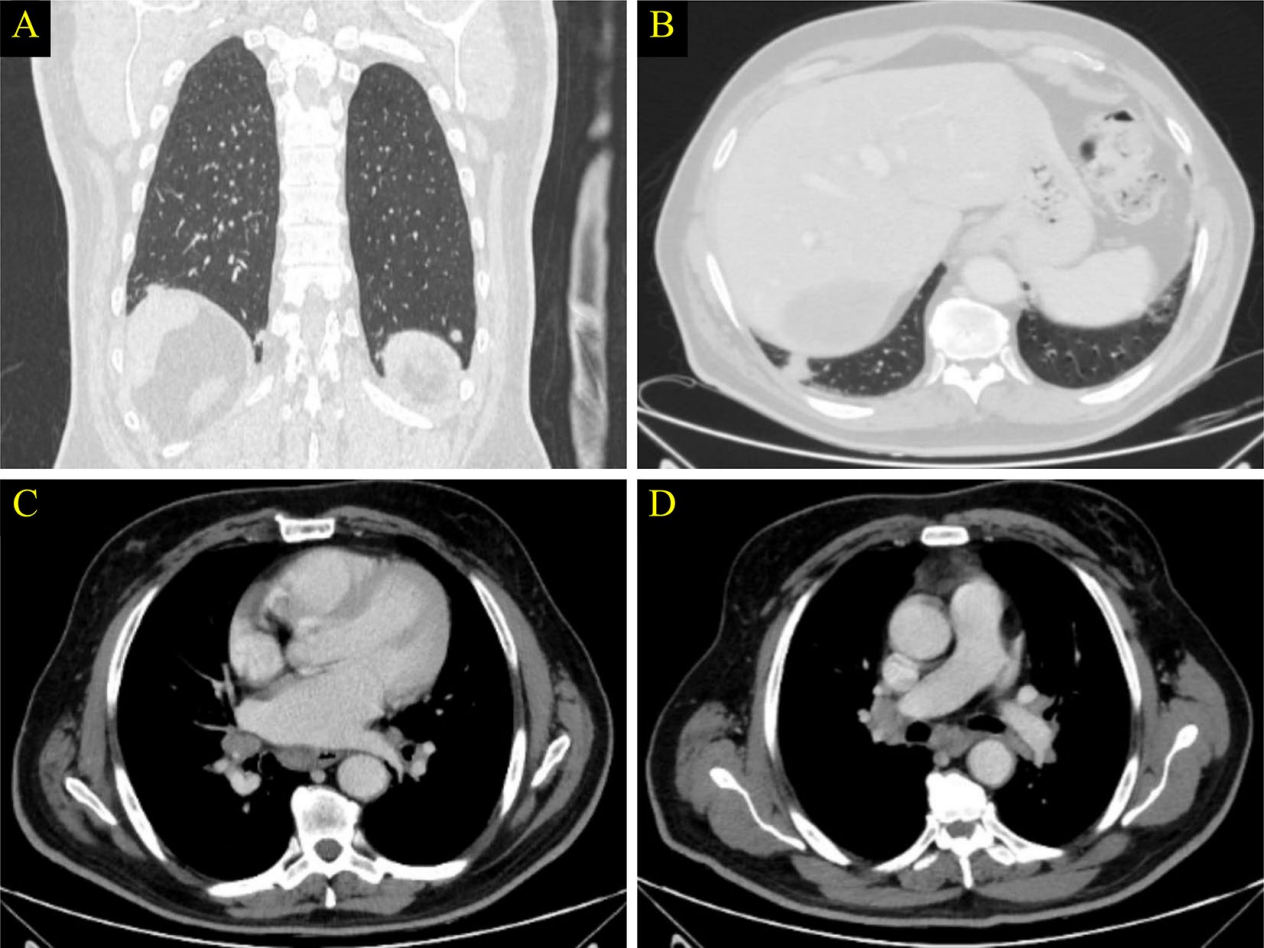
Selected coronal (A) and axial (B) cuts of CT scan with lung window demonstrating multiple bilateral subpleural lung nodules. (C and D) are selected axial CT scan at the level of the mediastinum showing multiple mediastinal and hilar lymph nodes (arrows)
